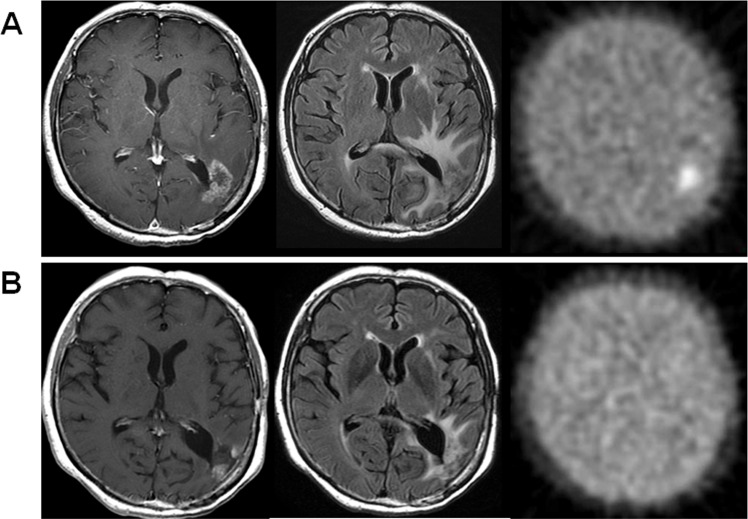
Representative cases of MRI-FMISO double responder (Case 10). The panel composition is same as Fig 4. After BEV treatment, contrast-enhanced lesion and FLAIR hyperintensity lesions were decreased. Simultaneously, FMISO accumulation was disappeared.
